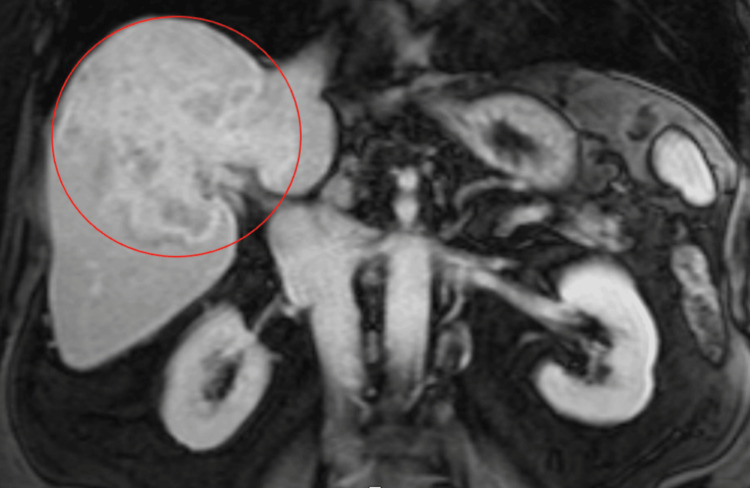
Coronal view of the MRI abdomen demonstrating a large mass in the central aspect of the right lobe of the liver measuring approximately 12.0 x 9.7 x 8.6 cm extending into the caudate lobe and abutting the IVC
